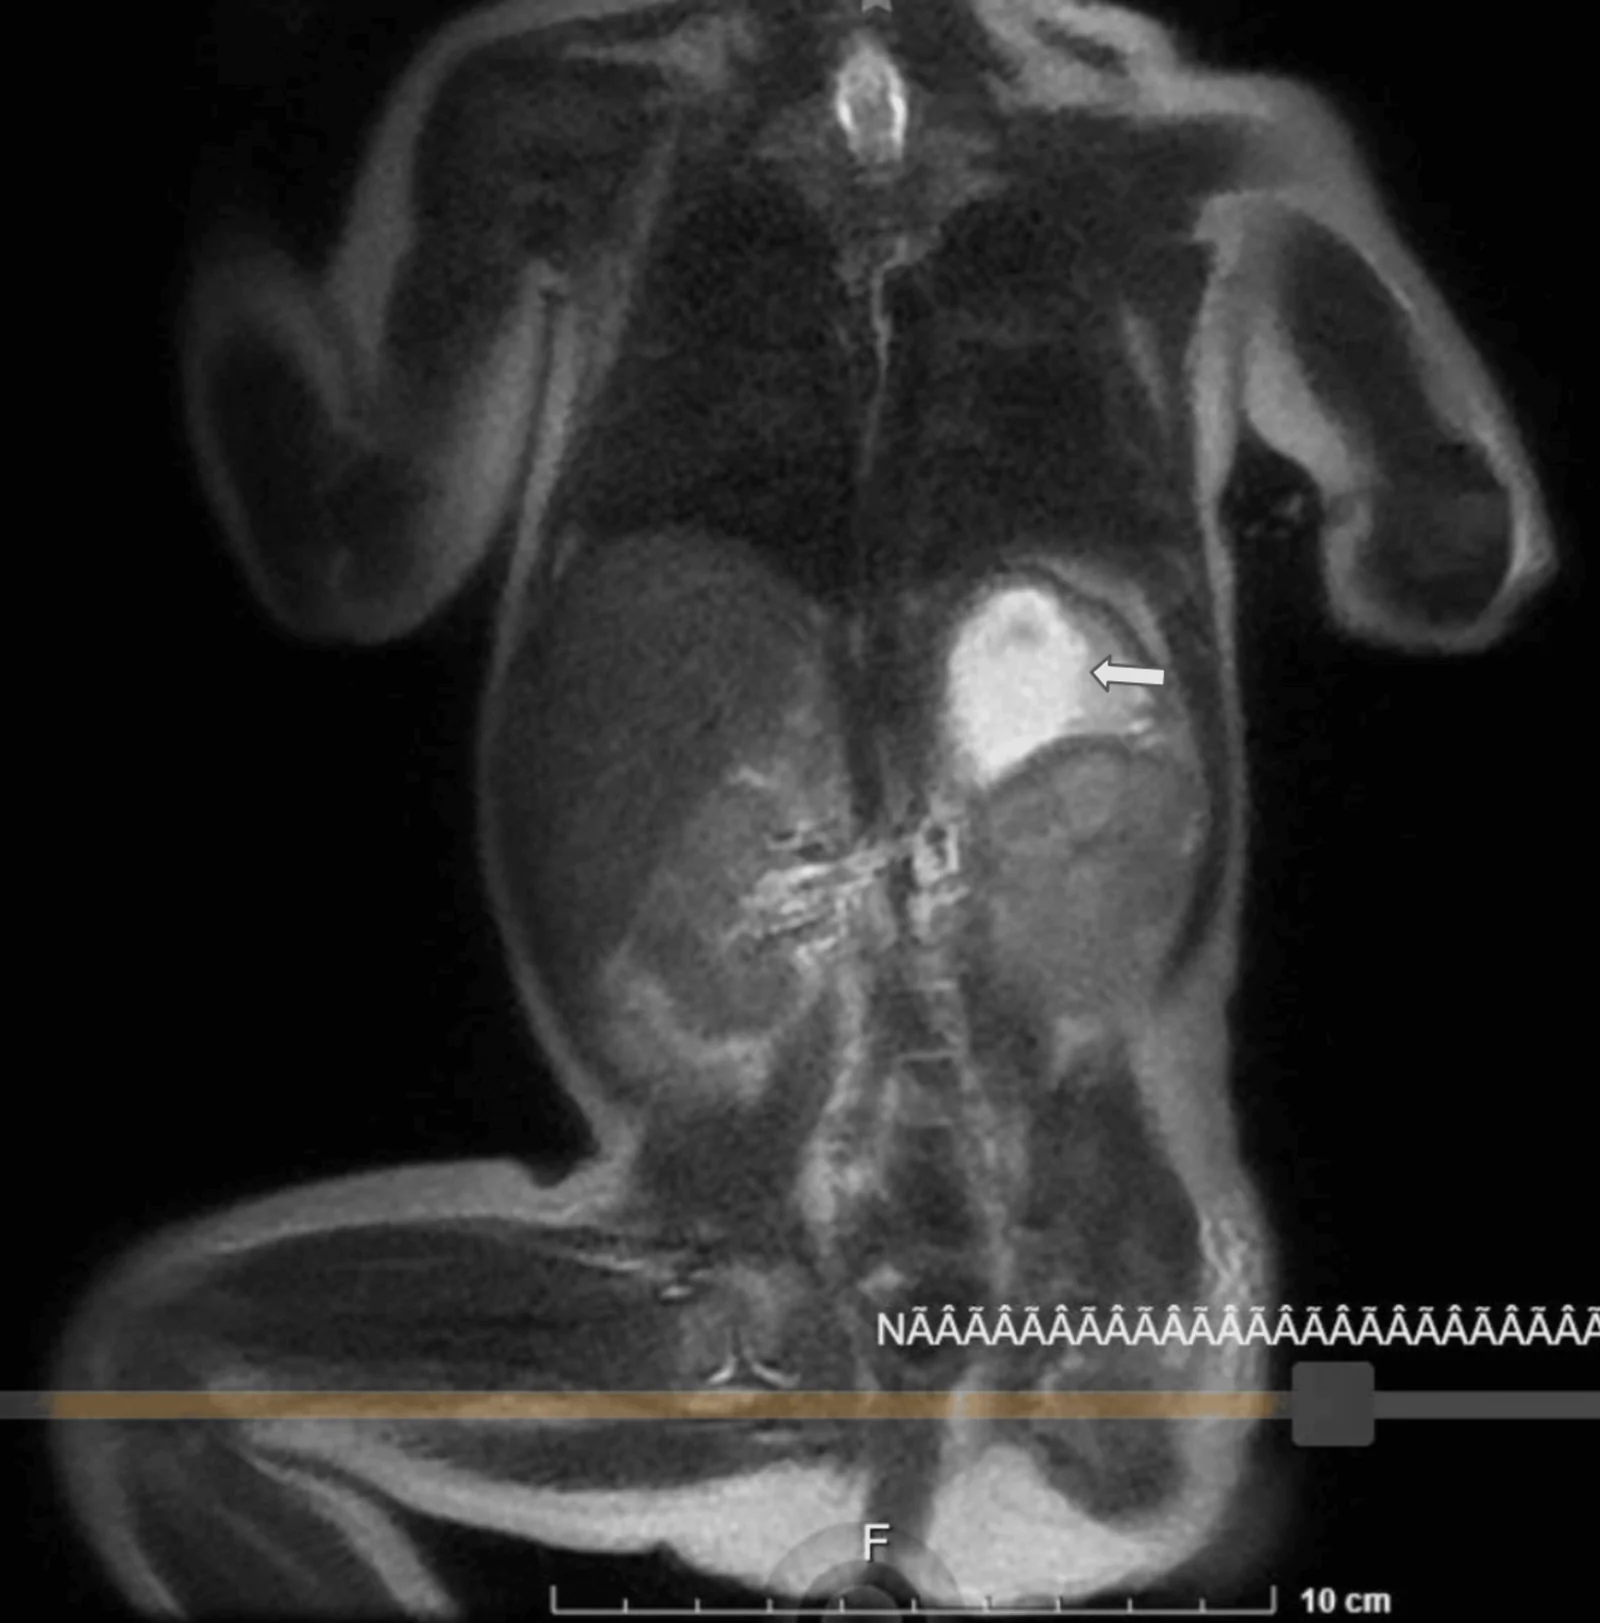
Left adrenal hemorrhage visualized on MRI as a 3.6 cm × 2.5 cm triangular shaped mass
